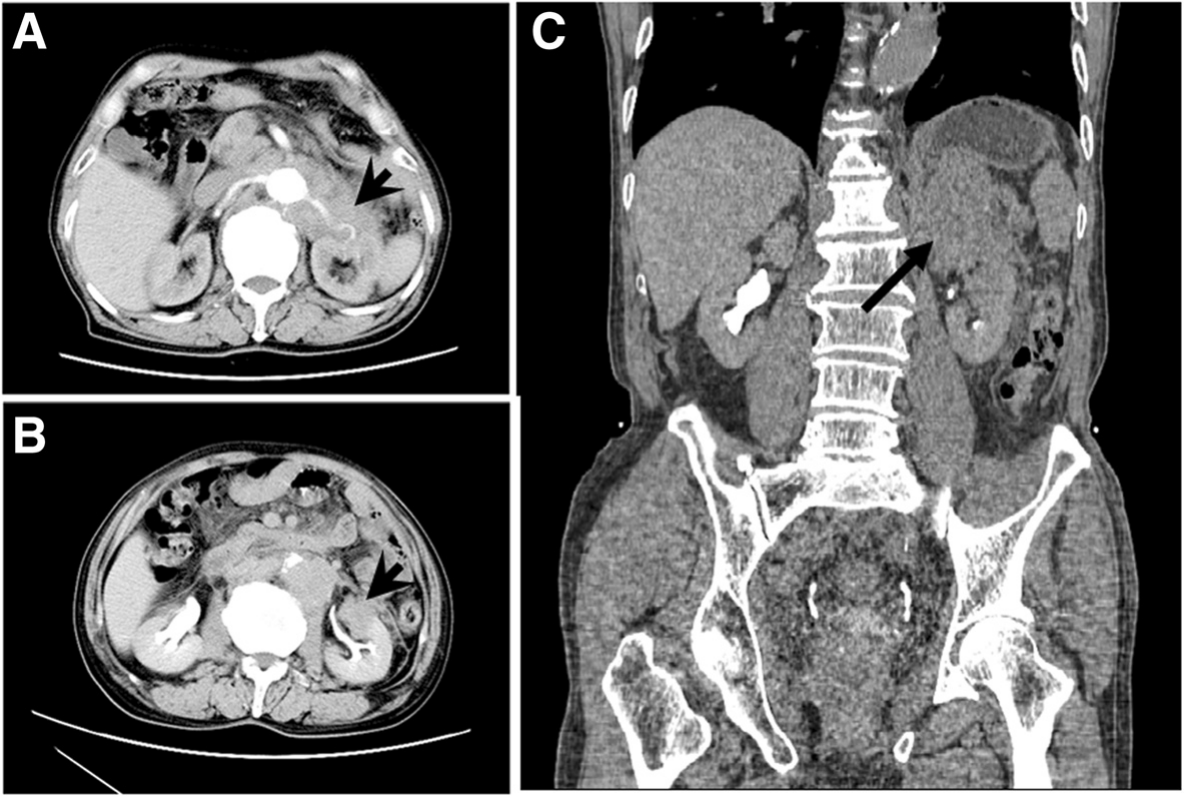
Computed tomography. a The arterial phase scanning image showing diffuse retroperitoneal mass wrapping the aorta and renal pedicle (*arrow head*), especially the left renal artery. b The excretory phase image showing the tumor (*arrow head*) located on the upper pole of the left kidney. c Coronal image revealed retroperitoneal mass with renal involvement (*arrow*)
